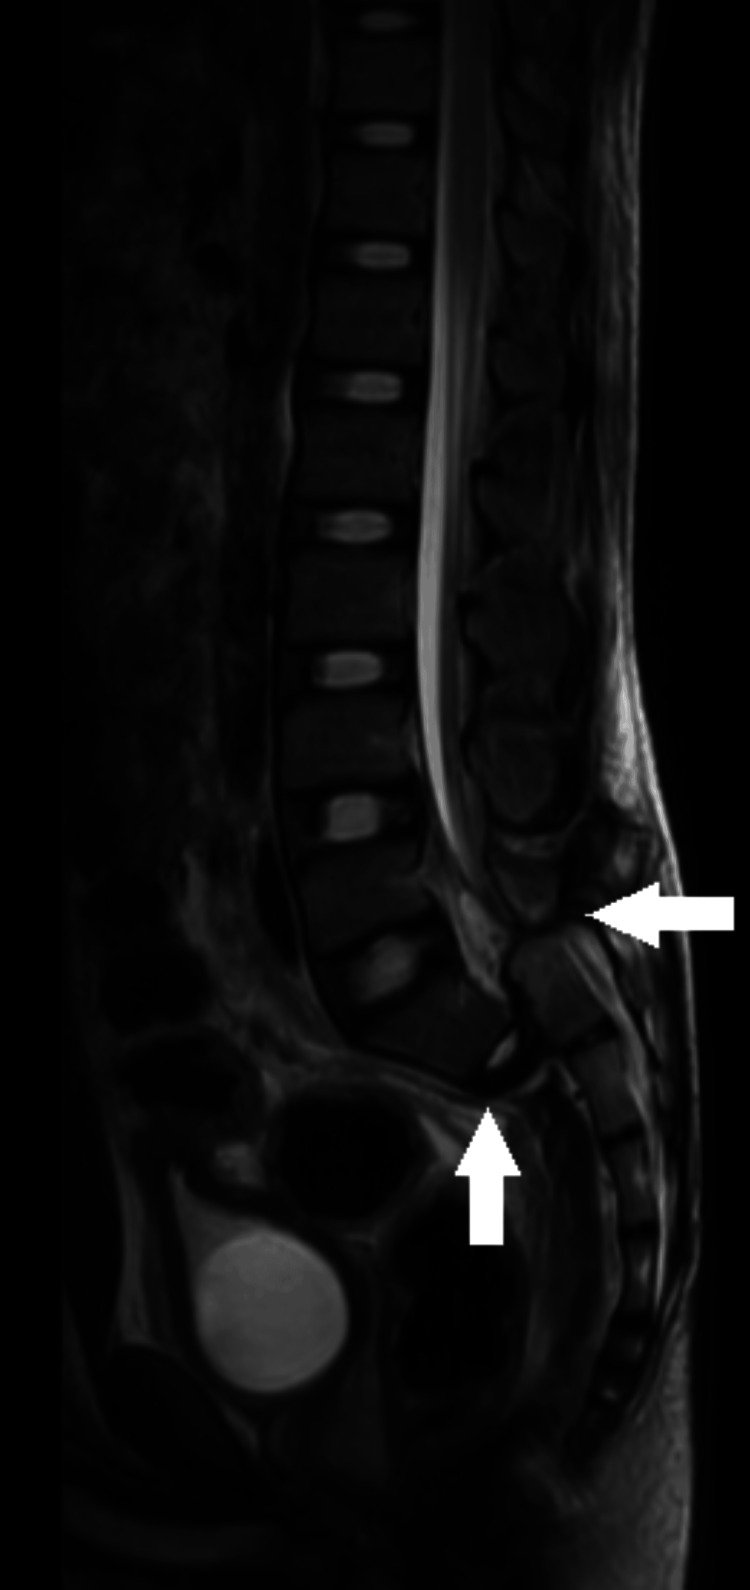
T2-weighted MRI image of the lumbar spine sagittal sequence demonstrating disruption of the anterior and posterior longitudinal ligaments with complete disruption of the L5-S1 intervertebral disc T2: transverse relaxation time
